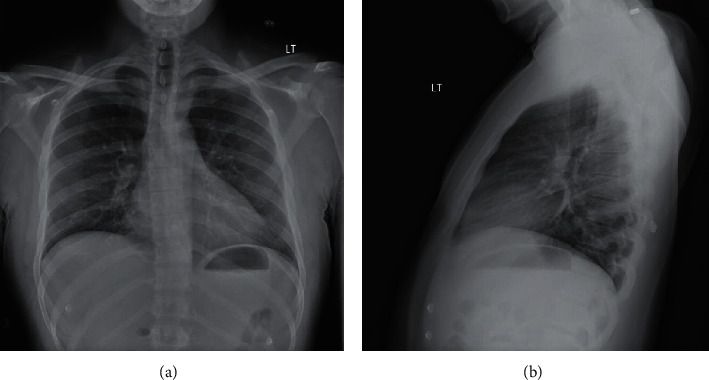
The patient's chest X-ray: (a) PA and (b) lateral. The lungs demonstrated no focal consolidation to suggest pneumonia and no evidence of pulmonary edema, pleural effusion, or pneumothorax. The cardiac silhouette was noted to be within normal limits, and no free air was detected below the diaphragms.
